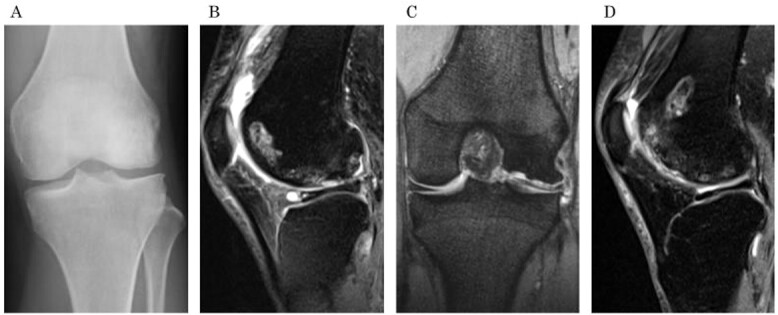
Preoperative radiographs and MRI. (a) An anteroposterior radiograph of the left knee shows the flattened lateral femoral condyle and irregular lateral joint surface. (b) A sagittal fat saturation T2-weighted image and (c) a coronal T2-weighted image show large osteonecrosis of the left lateral femoral condyle and lateral discoid meniscal tear associated with cyst formation. (d) A sagittal fat saturation T2-weighted image of right knee (contralateral knee) shows osteonecrosis of the lateral condyle similar to the left knee.
